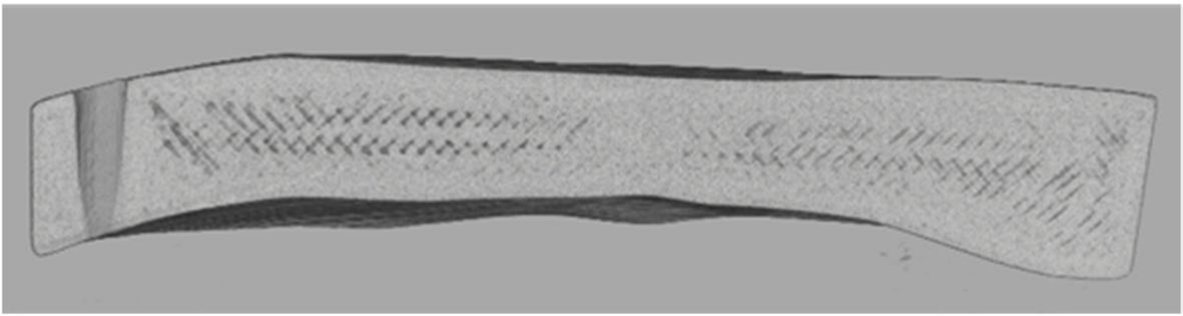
CT image for the selection of internal defects (PEEK)
